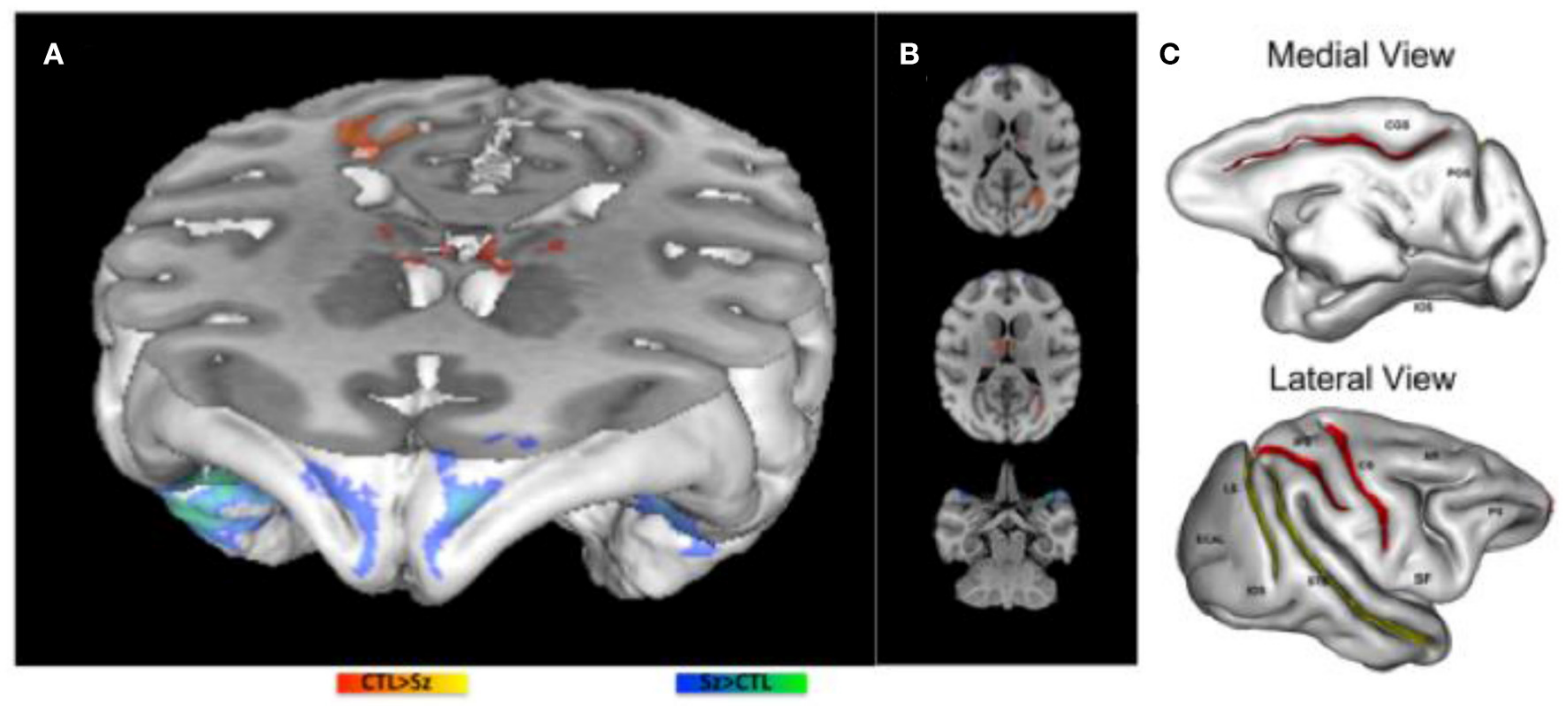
Morphometric MRI Analyses in the Epileptic Baboon. (A,B) demonstrate increases (blue) and decreases (red) in gray matter concentration in epileptic baboons compared to controls. (C) Demonstrate significant decreases (red) and marginally significant decreases (yellow) in sulcal areas of baboons with IEDs on scalp EEG compared to healthy controls [adapted from *Epilepsy Research*, references (35, 38), respectively].
